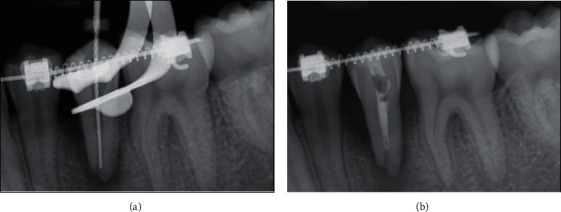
Initial endodontic treatment. (a) Periapical radiograph of working length of tooth #20. (b) Periapical radiograph of calcium hydroxide placement of tooth #20.
